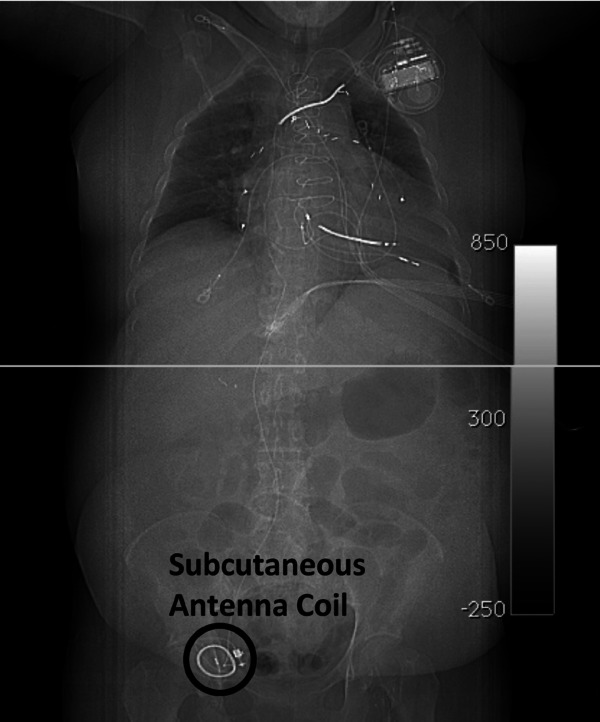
Scout film of chest and abdominal computed tomography scan demonstrating the placement of the ISL and concomitantly implanted pacemaker and defibrillator leads.
